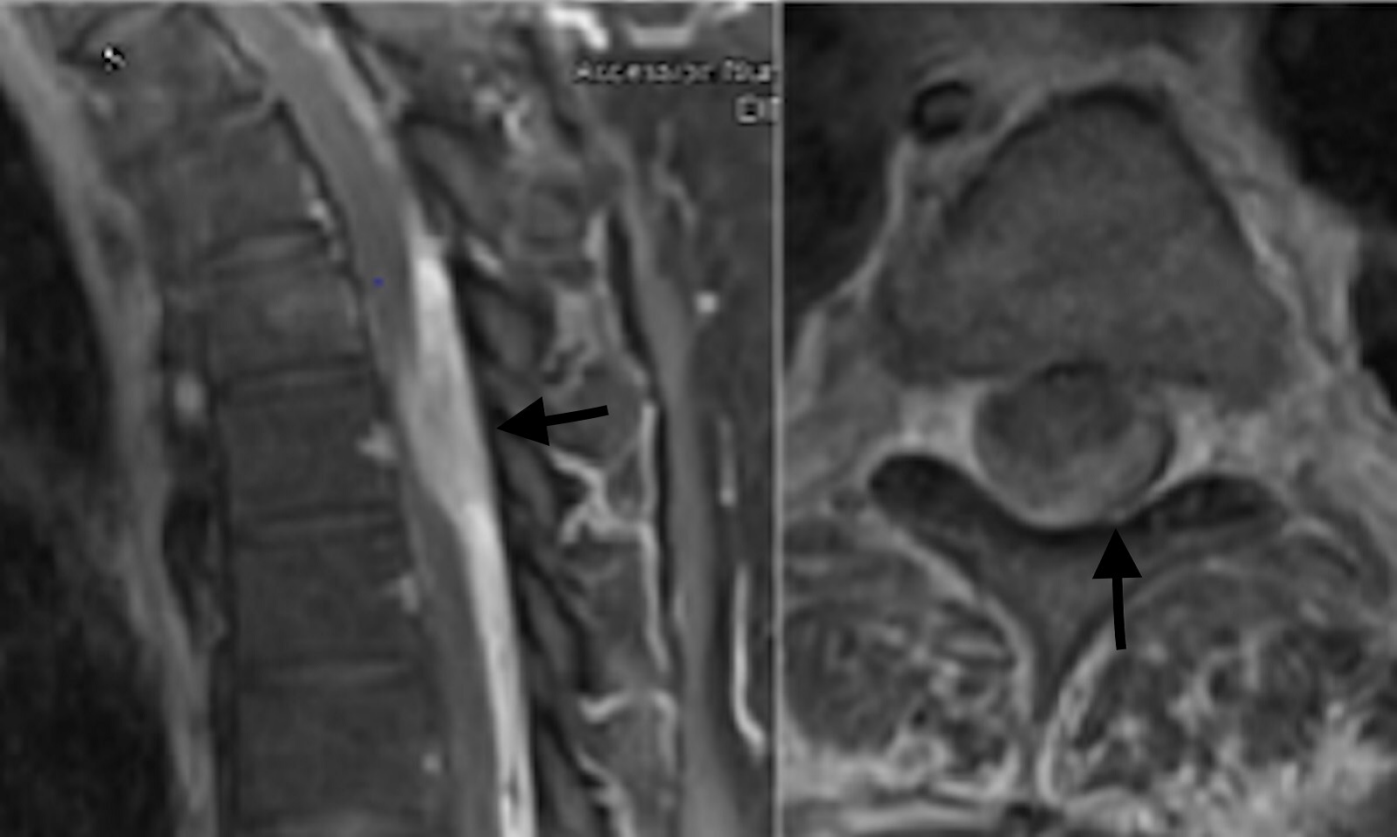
Thoracic T1-weighted magnetic resonance imaging (MRI). Sagittal and axial T1 post-contrast images revealing an enhancing extramedullary appearing lesion (black arrows).
